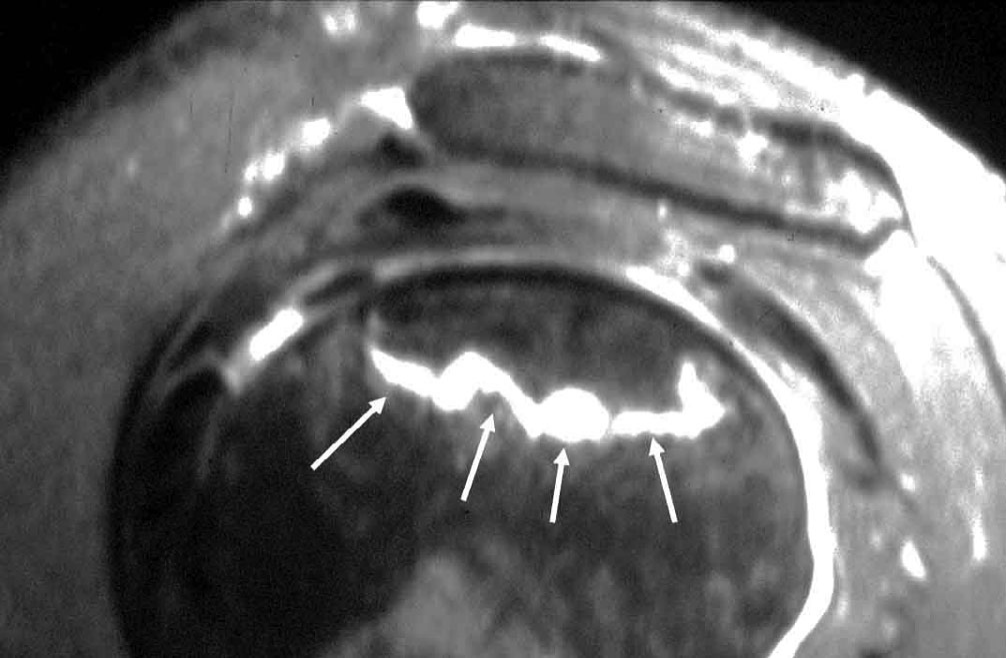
Area of high‐intensity signal on T2‐weighted images of osteonecrosis (arrow) on MRI.
